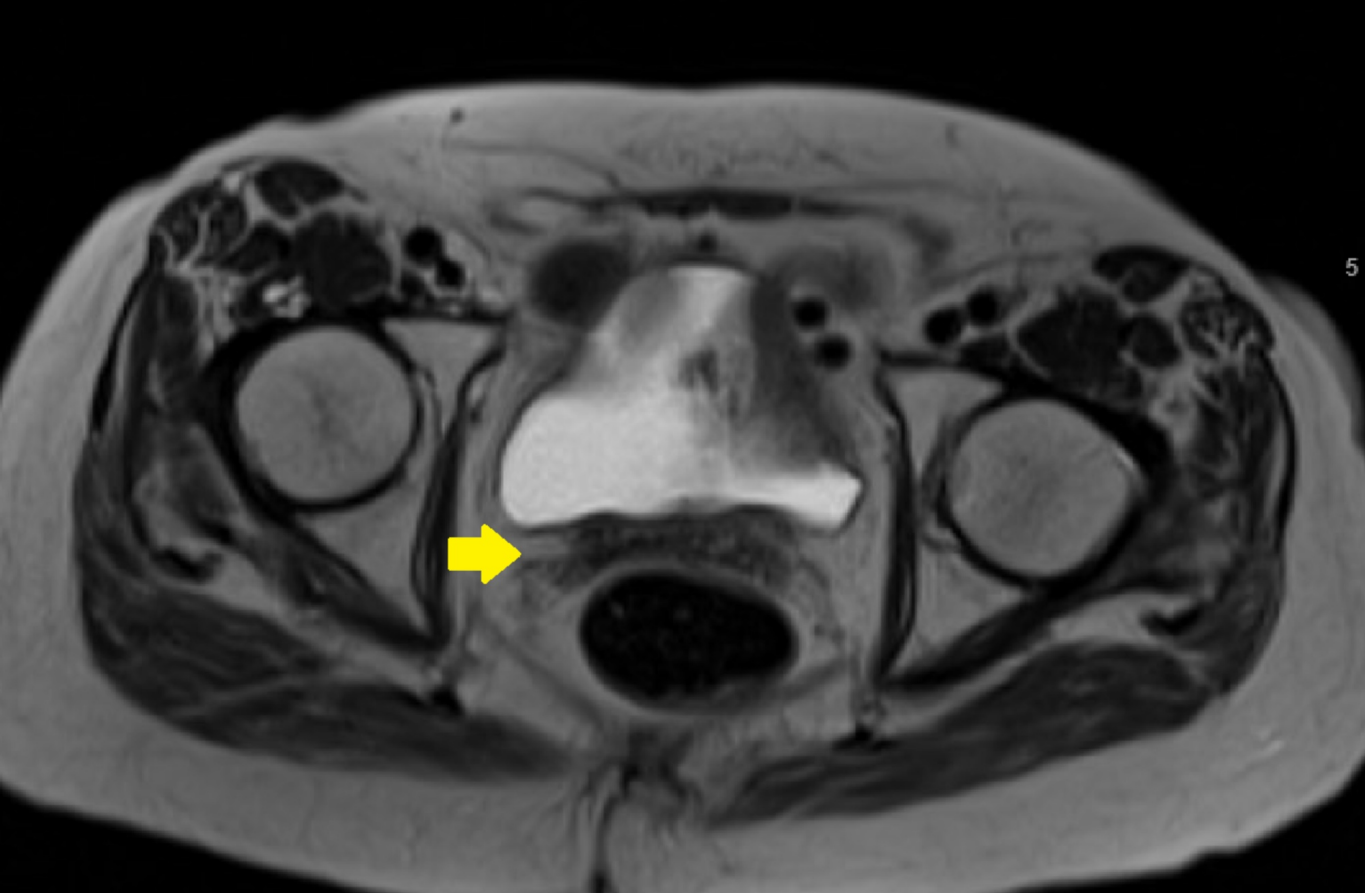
One-month post-treatment axial T2 magnetic resonance image
